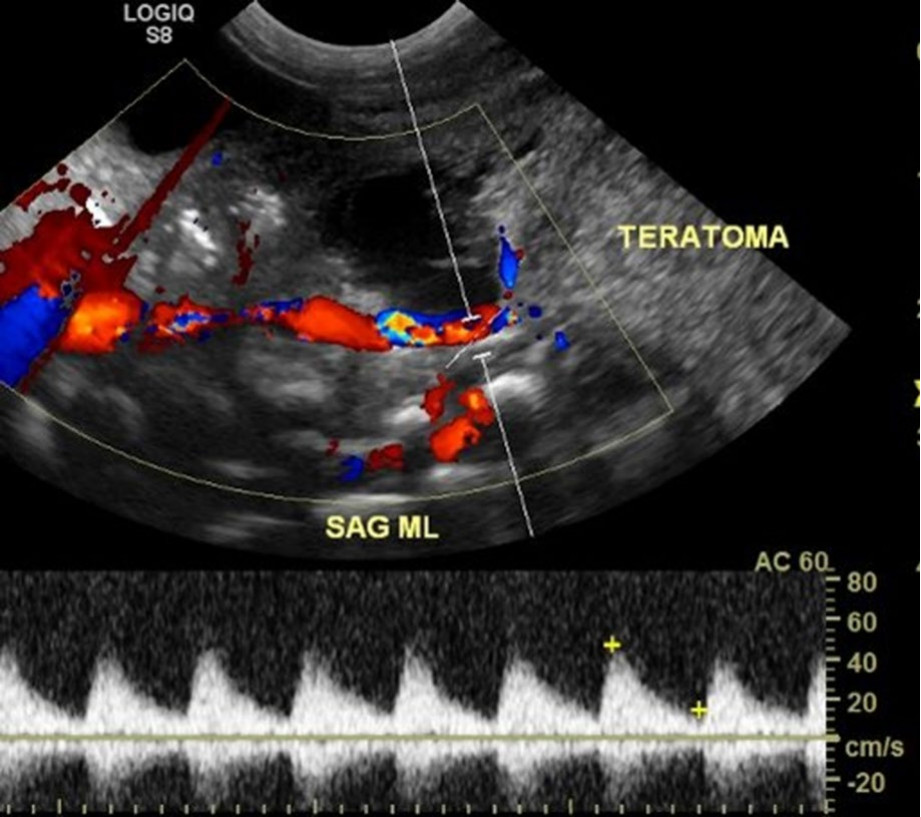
A pre-operative pelvic ultrasound was performed revealing a tiny distal vessel arising from the aorta appearing to be going in the direction of a solid and cystic lesion anterior to the sacrum, thought to be a teratoma
